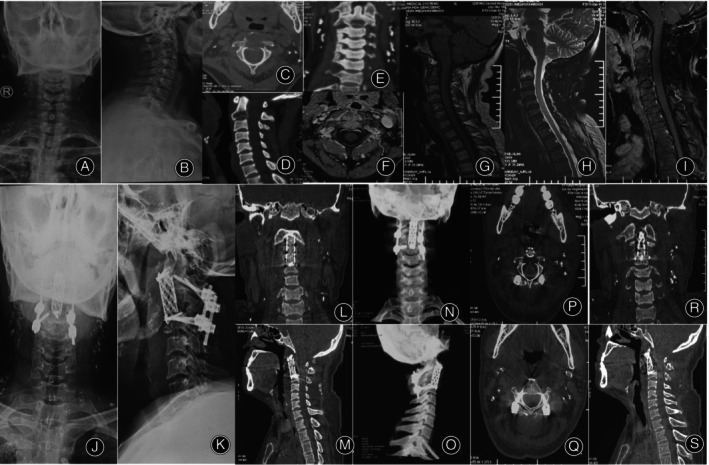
Female, 50 years old, with lung cancer metastasis to the bones. (A,B) Preoperative x‐rays showing lesions in the C2 vertebra. (C–E) Preoperative CT scans revealing bone destruction in the C2 vertebra, posterior vertebral bone defect, and spinal cord compression. (F–I) Preoperative lumbar spine MRI demonstrating C2 vertebral bone destruction, formation of soft tissue tumor around, and compression of the dural sac. (J,K) The x‐ray taken 1 week postoperatively showed that the cervical pedicle screws and the titanium cage were stable. (L–O) The CT scan 1 week postoperatively showed adequate spinal canal decompression, with the titanium cage and pedicle screws remaining stable. (P–S) The CT scan 6 months postoperatively showed no significant tumor recurrence in the surgical area, and the titanium cage and pedicle screws remained stable. CT, computed tomography; MRI, magnetic resonance imaging.
